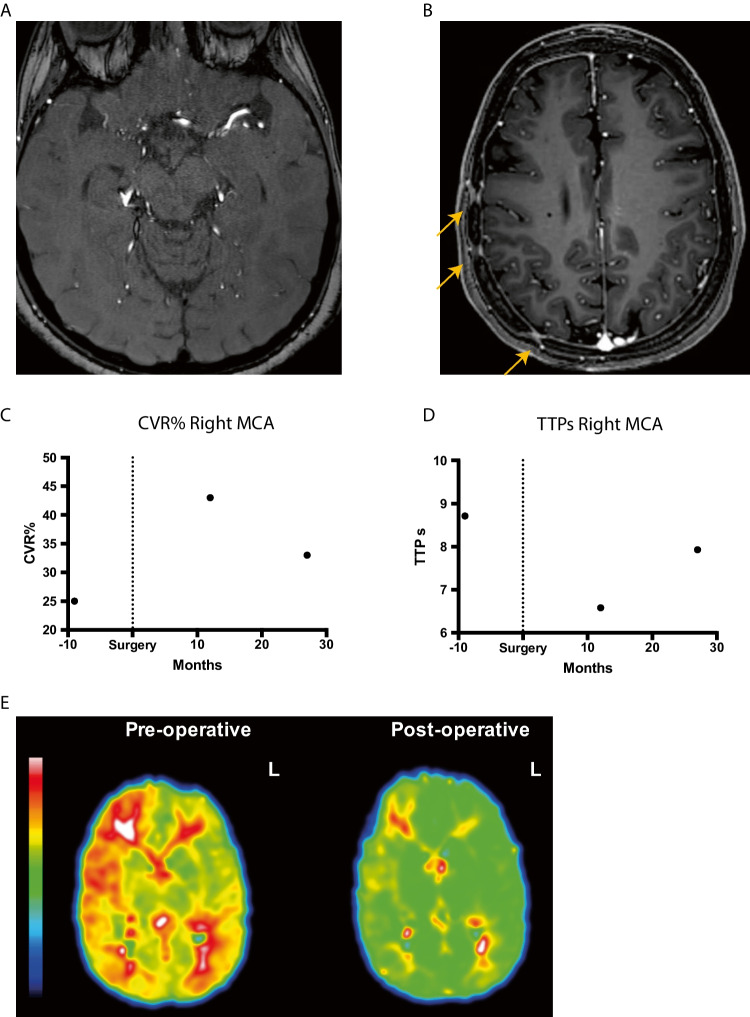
Patient 2 with right-sided MMS changes due to NF1. A Pre-op MRA showing occlusion of the right middle cerebral artery. B Post-op MRI showing contrast enhancement in multiple burr holes indicating ingrowth of blood vessels after MBH surgery. C Pre-op CVR was 25%, and 1 year later, it had increased to 43%. Second follow-up at 27 months showed a CVR decline to 33%, but no new symptoms. D and E Pre-op time-to-peak pre-op was similarly increased to 8.71 s, followed by a decrease to 6.58 s 12 months after surgery
